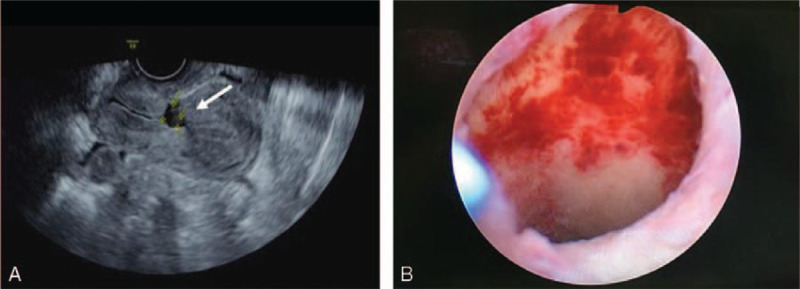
Diagnosis of CSD. A. Transvaginal ultrasound image of CSD: a wedge-shaped distortion in the integrity of the uterine incision scar was confirmed as CSD (white arrow). B. Hysteroscopy showed the “isthmocele” in the superior third of the cervical canal.
